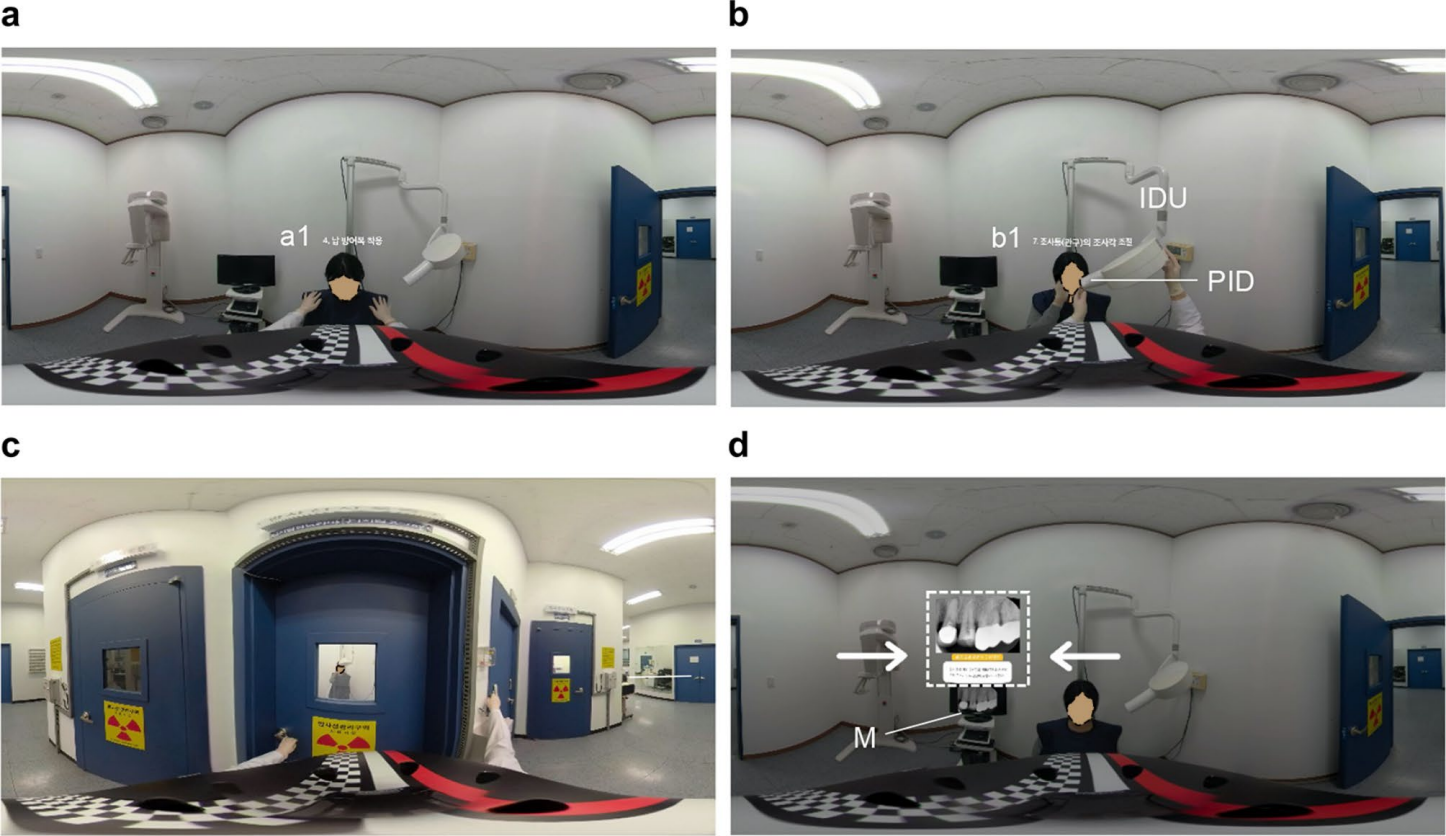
360° video according to the sequential dental radiography process. (a) Preparatory steps to help patient put on lead apron. (b): Step of manipulating the position indicating the device (PID) of the intraoral dental X-ray unit (IDU). (c) Steps taken outside the dental X-ray room. (d): Guide for checking on dental radiograph (white dotted box) and dental radiograph results on the monitor (M). a1: The sentence “a1” stands for “Put on lead apron” in Korean. b1: The sentence “b1” stands for “Manipulation of PID” in Korean
